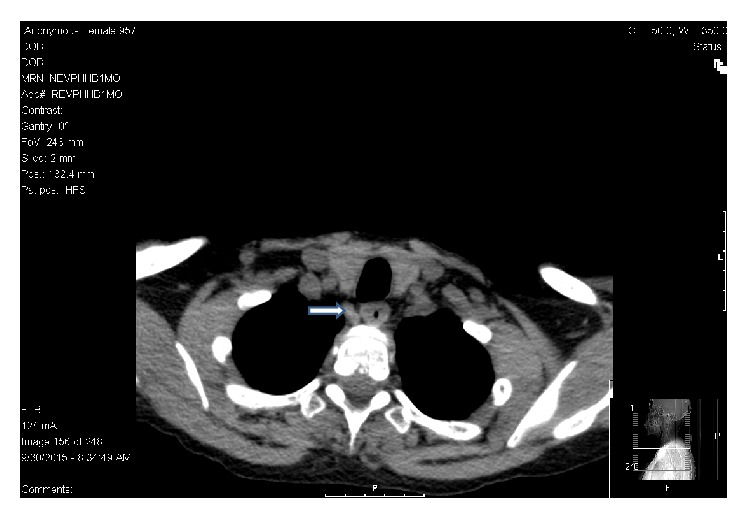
CT scan of the neck without contrast showing a soft tissue mass posterior to mid pole of the right thyroid lobe (denoted by arrow).
